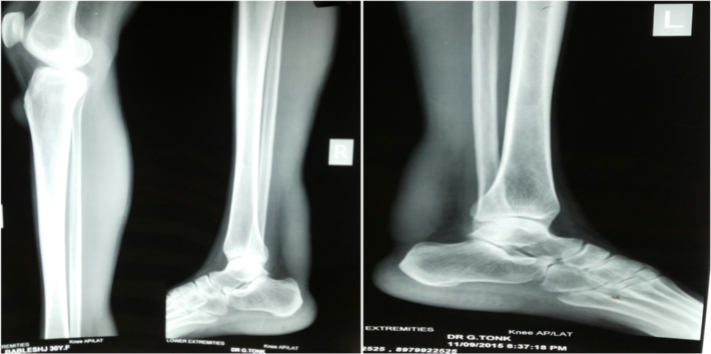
X-ray showing soft tissue thickening of bilateral tendo-Achilles
